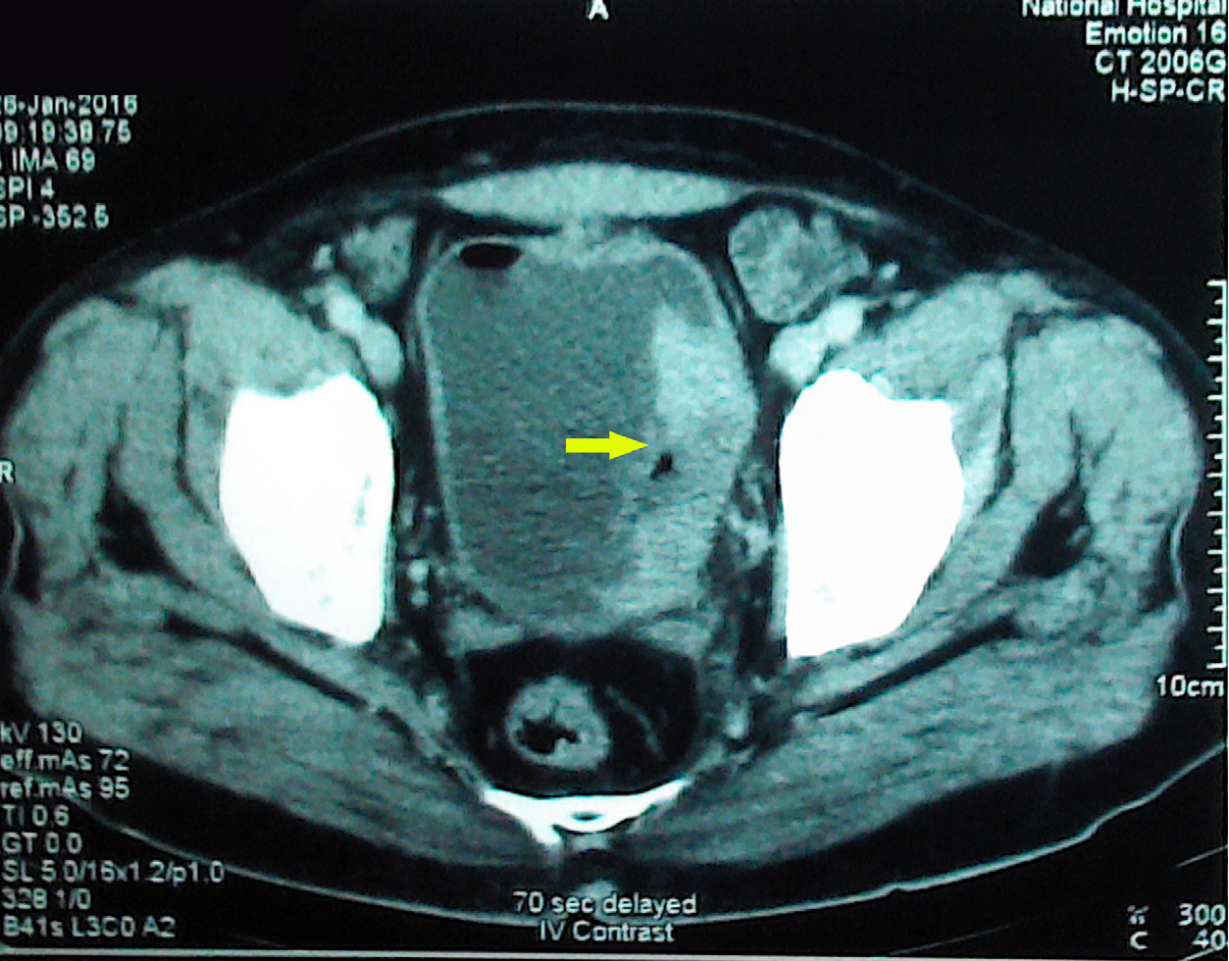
CT scan of the abdomen and pelvis showing a homogenously enhancing mass lesion in the left inferior lateral wall of the bladder measuring 5 × 4 × 4 cm, involving the left vesicoureteric junction.
